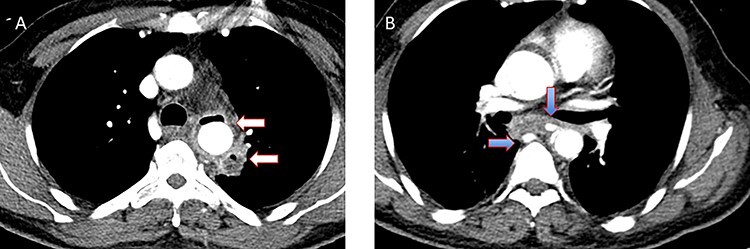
(A) CT study with contrast showing gas adjacent to the descending thoracic aorta with thickened soft tissue around the esophagus. (B) Vascular contrast within the esophagus and projecting outside the aorta forming ‘pseudoaneurysm’ or penetrating aortic ulcer.
